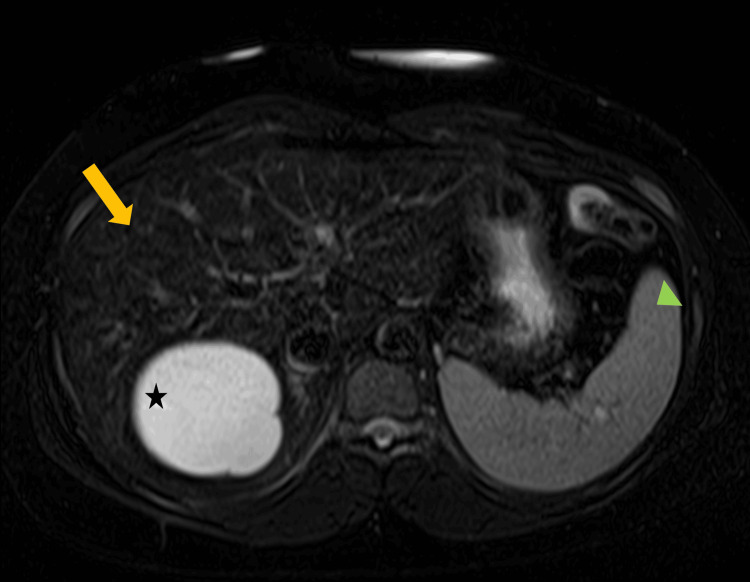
MRI liver protocol: radiographic hepatic siderosis Axial contrasted MRI (T2-weighted gradient echo) in a patient with iron overload secondary to PCT and HH. Contemporary MRI technology can accurately visualize and quantify excess hepatic iron. The visceral iron deposition causes a magnetic susceptibility artifact and T2 signal loss, resulting in decreased intensity on MRI (the darker the liver, the higher the iron content). Gradient in/out-phase and gradient echo MR sequences provide an important diagnostic clue of iron overload in patients presenting with PCT and elevated transaminases. In our patient, MRI demonstrates a typical finding in HH, with a sharp contrast between the dark liver (yellow arrow) and the "white" spleen (green arrowhead). The figure also shows an incidentally discovered 8.4 cm simple cyst in the upper pole of the right kidney (black star) MRI, magnetic resonance imaging; PCT, porphyria cutanea tarda; HH, hereditary hemochromatosis; MR, magnetic resonance
